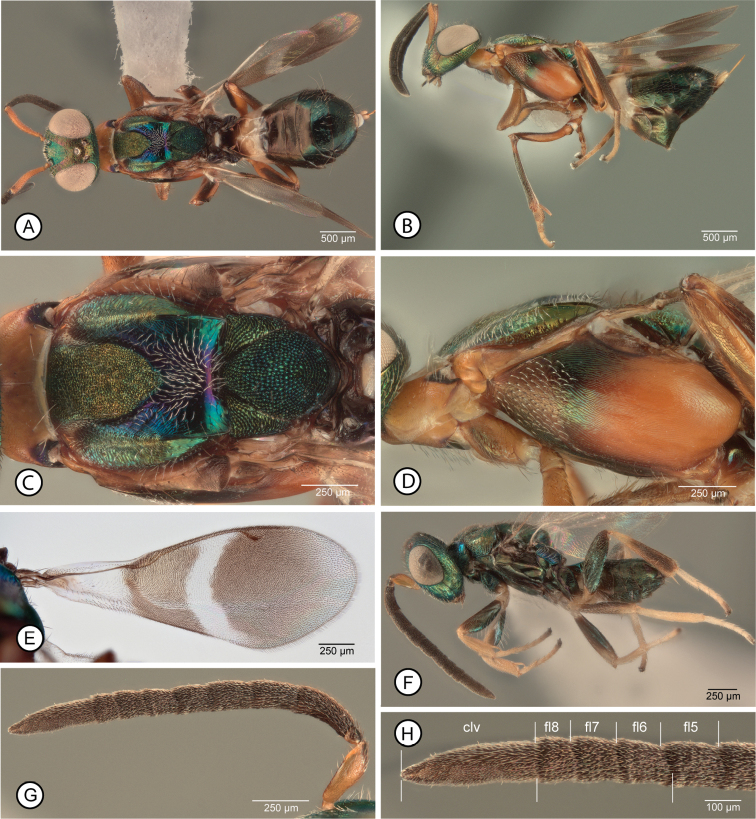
Anastatusjaponicus*A–E female: A dorsal habitus (6) B lateral habitus (4) C mesosoma in dorsal view (6) D same in lateral view (4) E fore wing (5). F–H male: F lateral habitus (25) G antenna (25) H clava and apical three funiculars (25) (three lower bars indicate length of clava compared to combined length of apical funiculars). Abbreviations: clv = clava, flx = flagellomere number.
